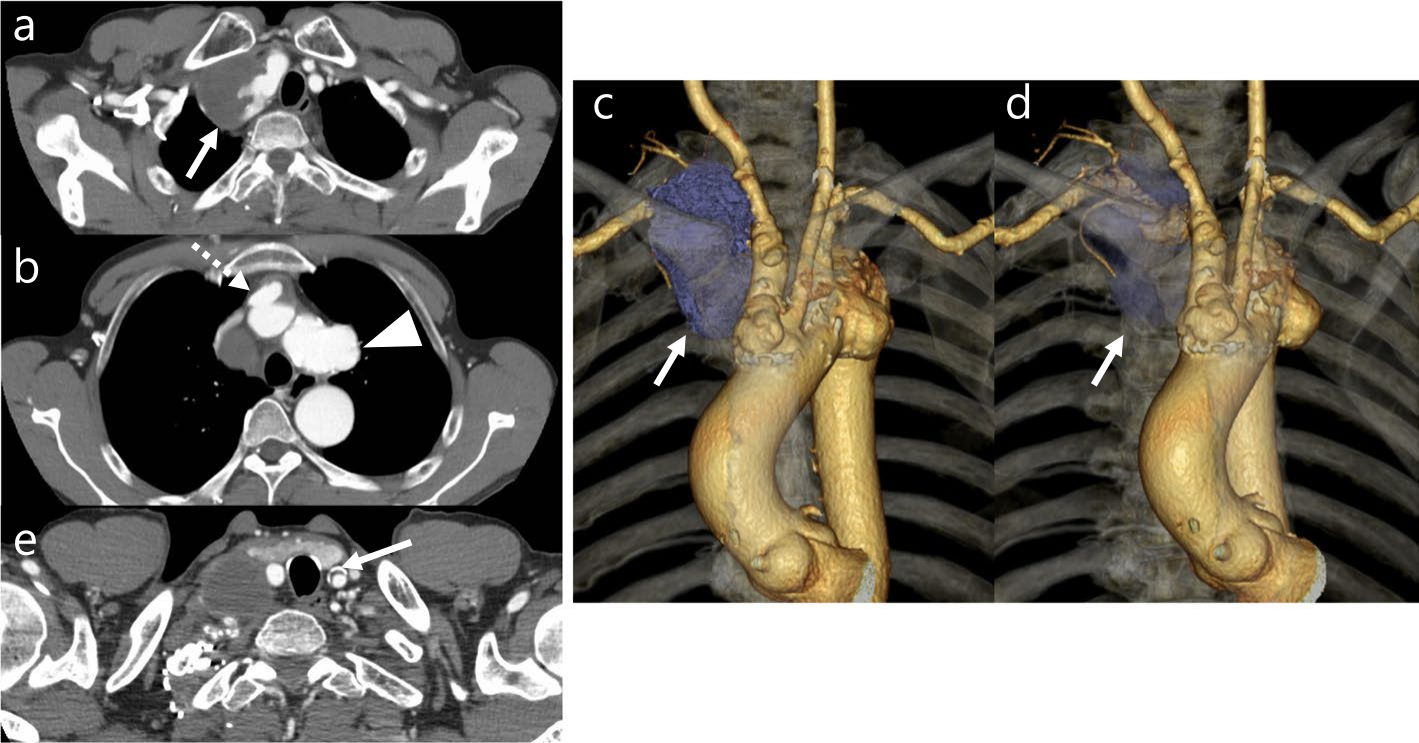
a, b Preoperative computed tomography scan revealing aneurysms of right subclavian artery (solid line) and brachiocephalic artery (dotted line) and saccular aortic arch aneurysms (arrow head). c, d Preoperative three-dimensional computed tomography scan revealing right subclavian artery aneurysm (blue color lesion, arrow head). e Preoperative computed tomography scan revealing moderate plaque in the left common carotid artery (solid line)
